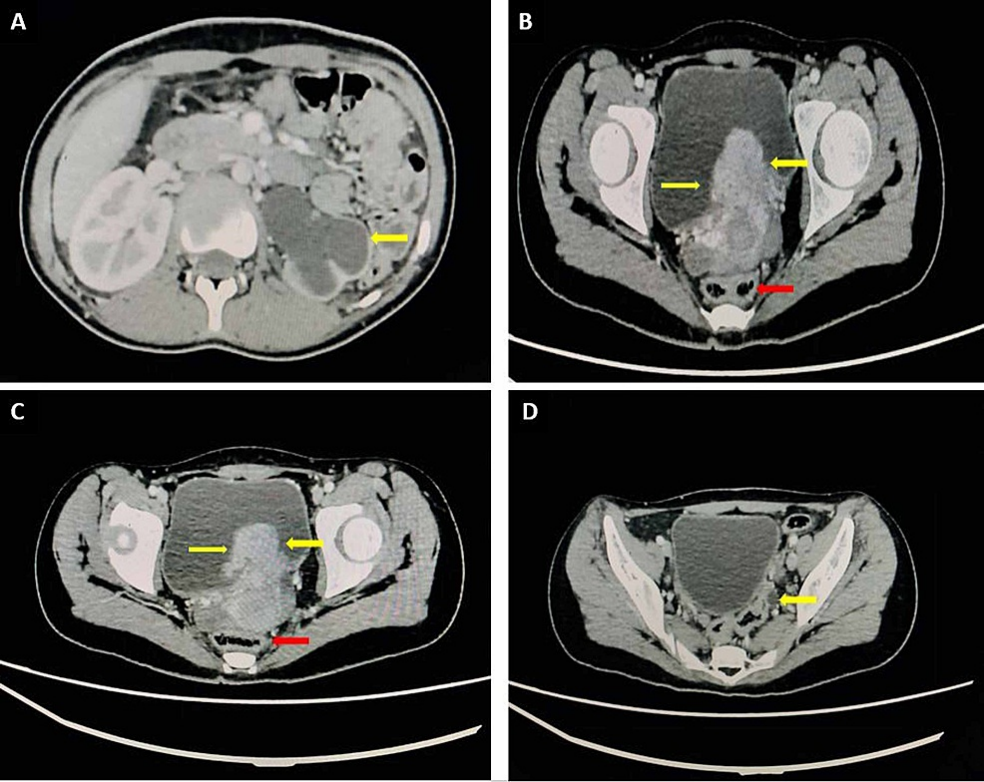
Contrast-enhanced computed tomography (CT) of the abdomen and pelvis (A) Axial selected section at the kidneys level, demonstrating left-sided dilated pelvicalyceal system with thinning of the cortex (hydronephrosis). (B & C) Selected axial images demonstrating a large heterogeneously enhancing mass lesion (yellow arrows) arising from the left posterior wall of the urinary bladder with no obvious rectal (red arrows) infiltration. (D) Dilated distal left ureter (arrow).
